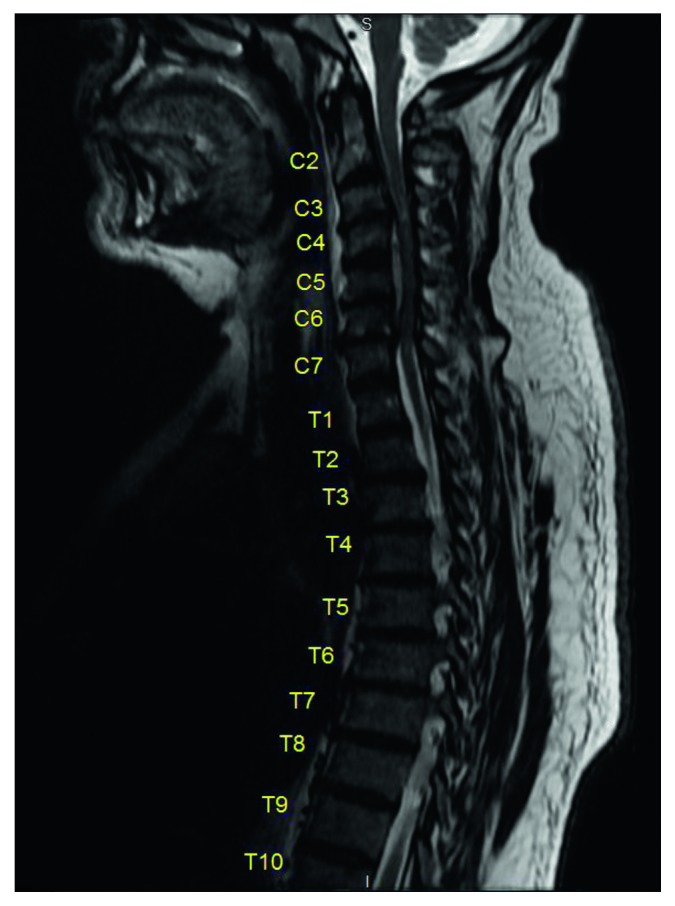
MRI with contrast of spine shows peripherally enhancing, approximately 1.2 × 4.6 cm, T2 hyperintensity/T1-hypointense collection that is located along the posterior vertebral bodies C4–C6.
